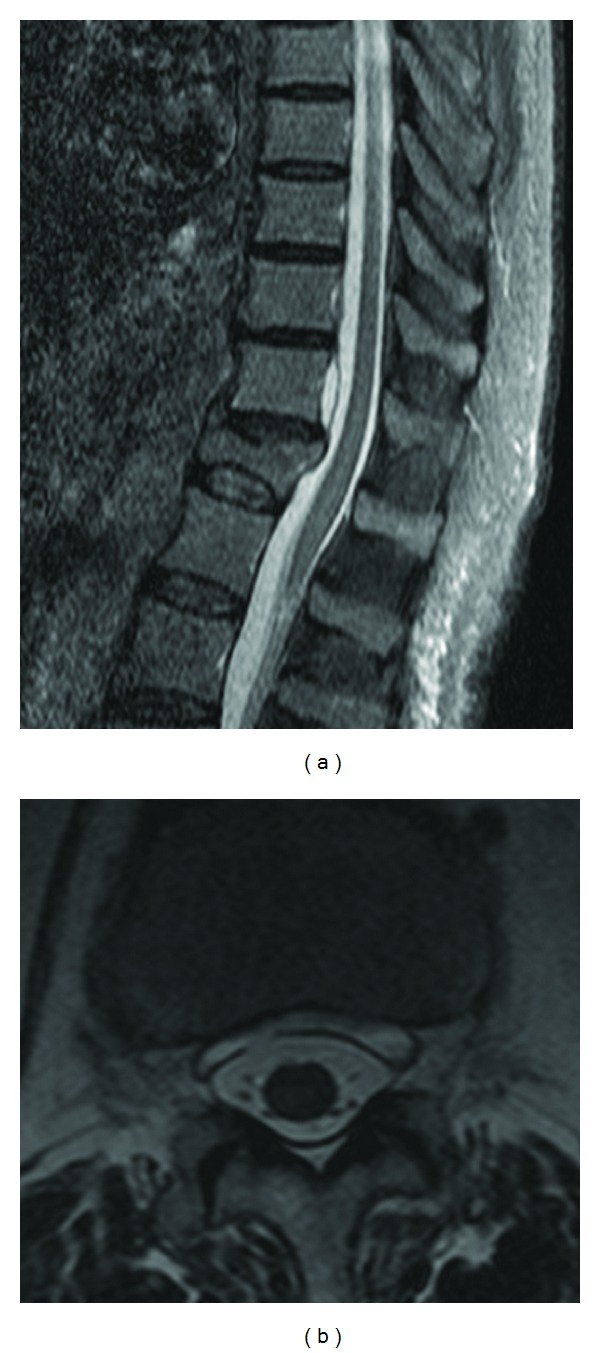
A sagittal STIR MRI (a) reveals an anterior subdural haematoma at T11 level with adjacent fracture and collapse of the 12th thoracic vertebral body, probably due to trauma. In this T2-pondered axial slice (b) there seems to be continuity between the haematoma and the subarachnoid space.
